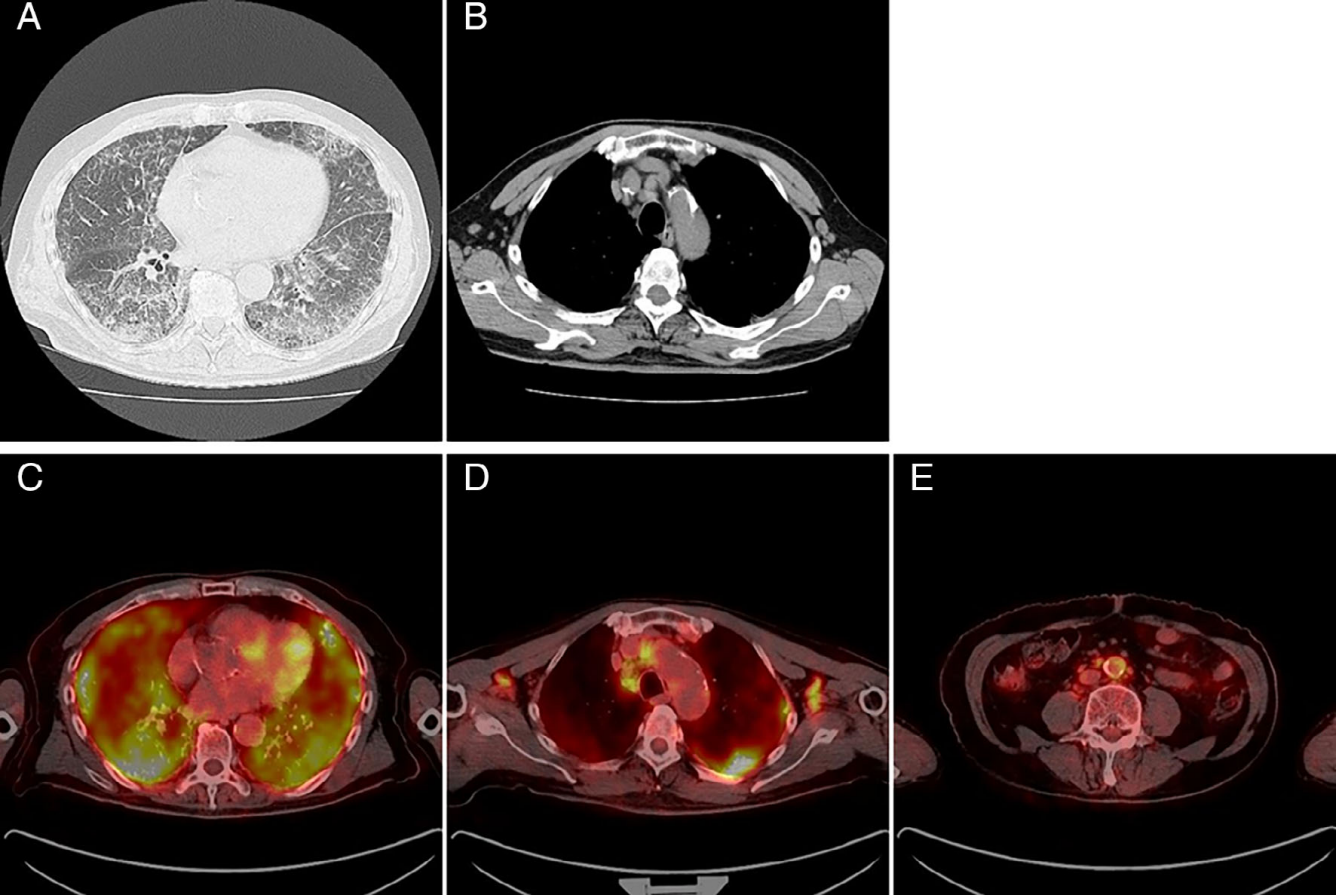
Chest computed tomography (CT) at the first consultation to our department showed non‐segmental reticular shadow and ground‐glass opacity predominantly spreading over the lymphatic tract, including the centrilobular and perilymphatic areas (A) accompanied by enlarged lymph nodes in the mediastinum and bilateral axillae (B). ^18^F‐fluorodeoxyglucose (FDG) positron emission tomography/CT showed significant FDG accumulation in the lung fields (C), the mediastinal, axillary (D), and para‐aortic (E) lymph nodes as well as abdominal aorta (E).
